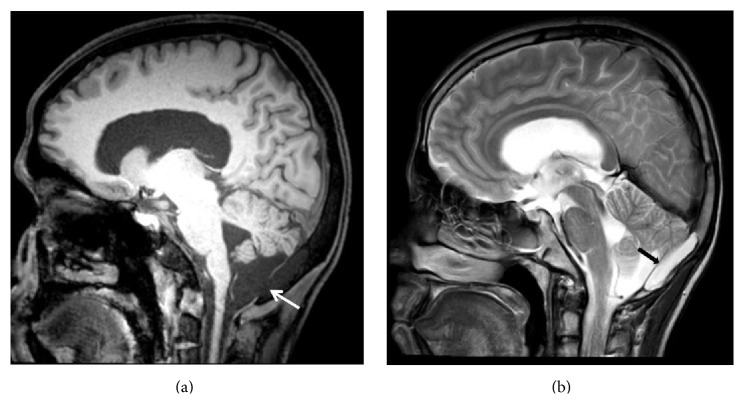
MRI brain (a) T1 sagittal and (b) T2 sagittal images showing intradiploic space expansion with CSF intensity lesion (black arrow) communicating with the cistern magna through a dural tear (white arrow).
